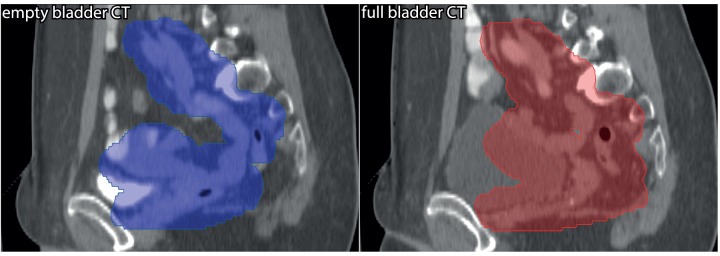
For a study patient, sagittal views of the empty bladder CT scan with the empty-to-half-full-bladder PTV (blue) projected on it (left), and of the full bladder CT scan with the half-full-to-full-bladder PTV (red) on the (right). The higher degree of concavity of the empty-to-half-full-bladder PTV increases the complexity of small bowel sparing in manual planning.
